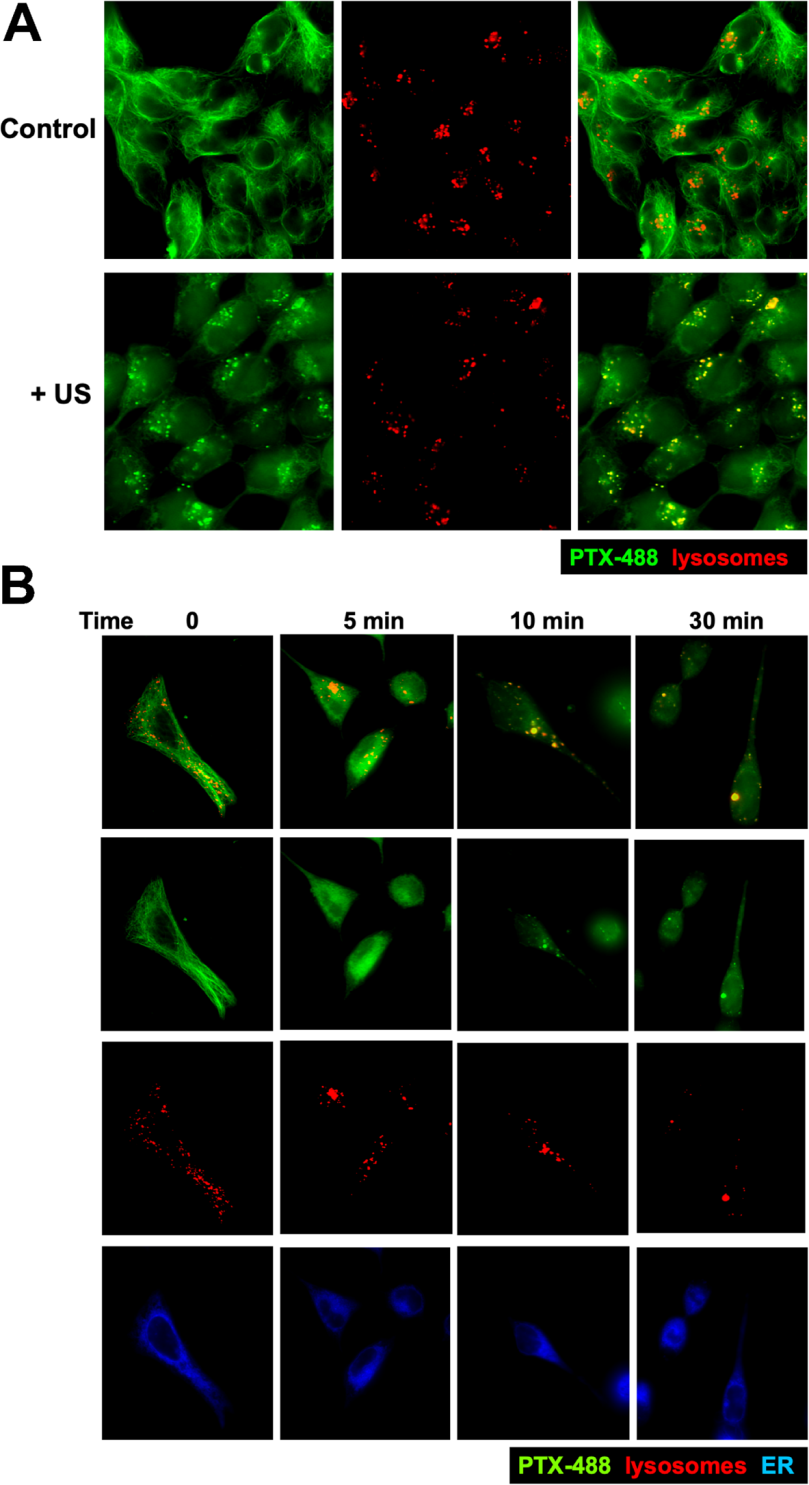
Re-localization of fluorescence labeled Taxol to lysosomes following ultrasound. A Breast and ovarian cancer cells were added with fluorescence labeled Taxol analog (488-PTX) (1 nM) and Lyso-marker for 60 min. The cells on tissue culture dish were exposed to ultrasound (+ US, 40 KHz, 1.0 W/cm^2^) for 5 min. Representative 488-PTX images were taken before (control) and after exposure to ultrasound. The signals from 488-PTX and Lyso-Marker were combined/overlaid. An example of A2780 cells is shown. B Cells incubated with fluorescence labeled Taxol analog (488-PTX) (1 nM), ER-Tracker™, and Lysomarker (BioTracker LYSO-TP Live Cell Dye) markers for 60 min before exposure to ultrasound for 5 min. After exposure to ultrasound at time 0 (no ultrasound), 5, 10, 30 min, the cells were imaged. Representative images of OVCAR5 cells are shown
